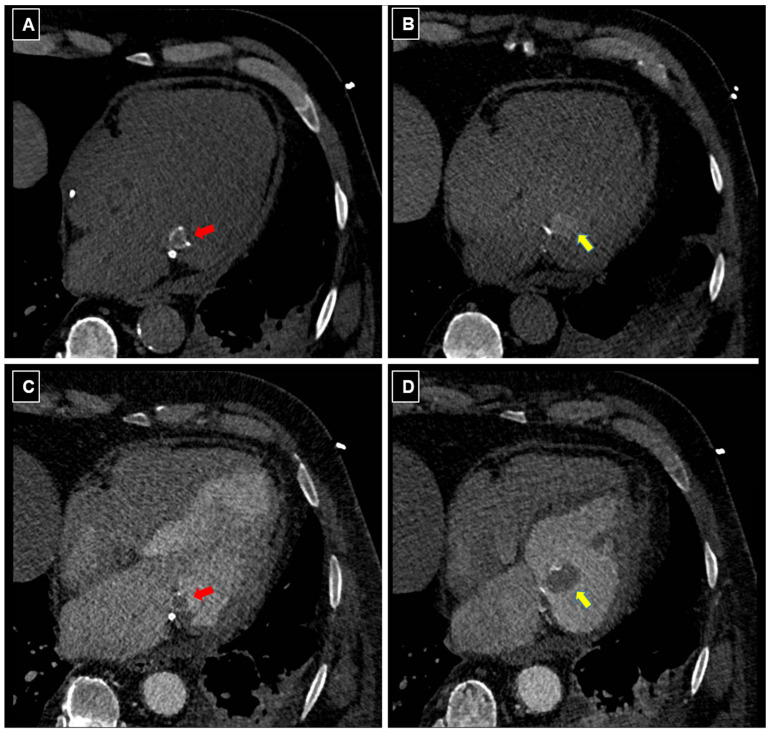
Computed tomography (CT) features of complicated CCMA. Baseline (A,B) and following contrast enhancement (C,D) trans-axial CT images.
